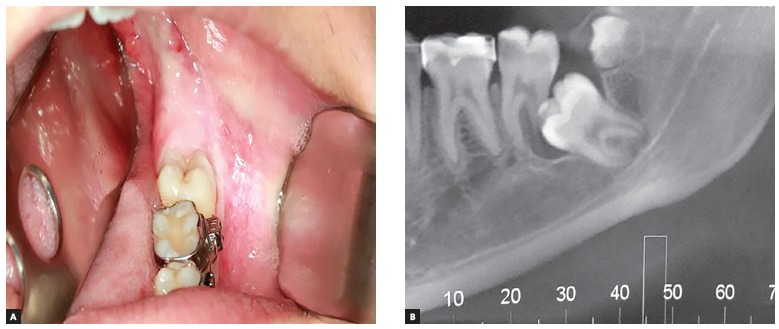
Pre-operative aspect, left posterior mandibular region: A) Clinical aspect; B) CT image, showing unerupted tooth #38 and the supernumerary tooth, as well as the root resorption of tooth #37.
